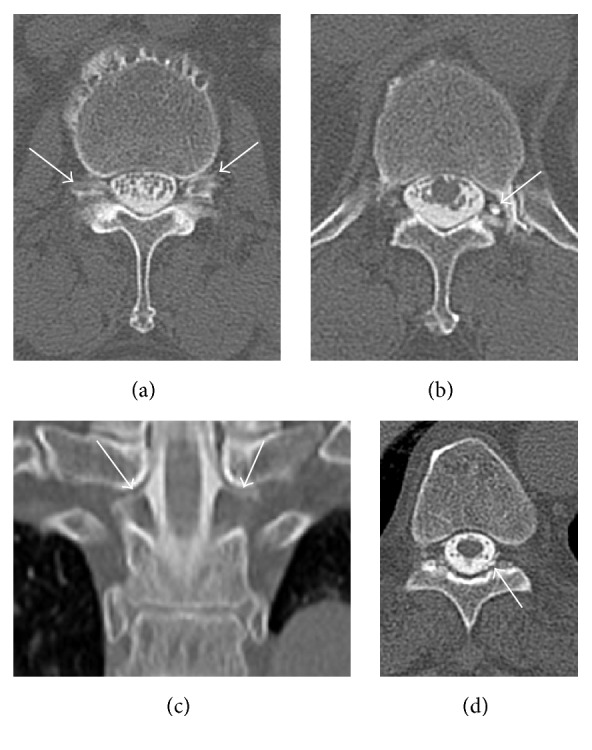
Computed tomography (CT) myelograms showing spontaneous spinal cerebrospinal fluid (CSF) leaks. (a) Bilateral CSF leaks (*arrows*) at L2-3 levels. (b) A contrast-filling nerve root sheath (*arrows*) at left T12-L1 level. (c) A coronal reconstruction image showing bilateral contrast-filling nerve root sheaths (*arrows*) at T1 level. (d) A small amount of contrast leak (*arrow*) in left T10 epidural space.
